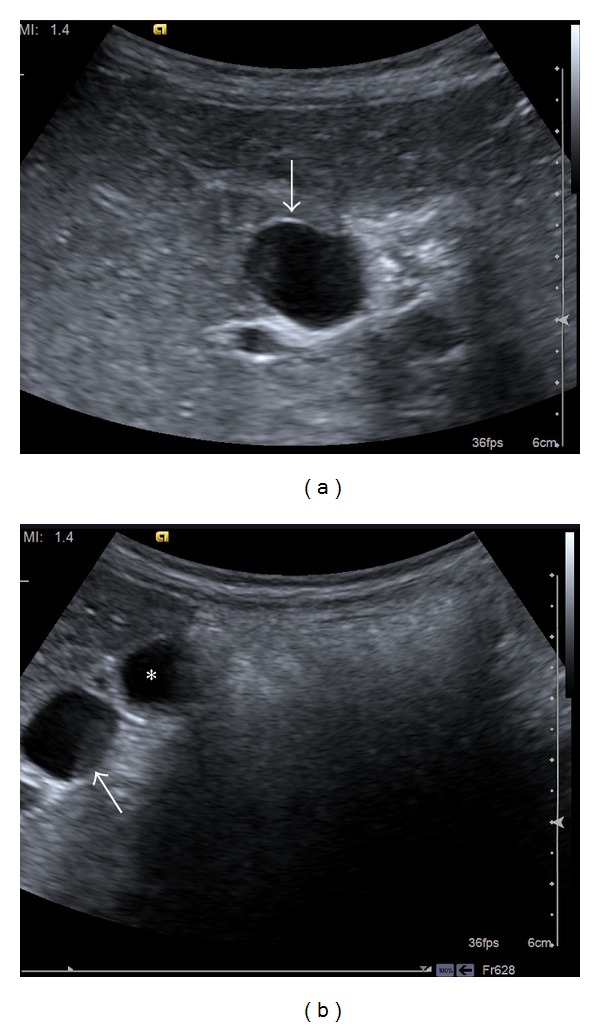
Ultrasound image of the cystic duct cyst: (a) the cystic duct cyst at the anterior aspect of the portal vein (white arrow); (b) the cystic duct cyst (white arrow) with the anteriorly located gall bladder (asterisk).
